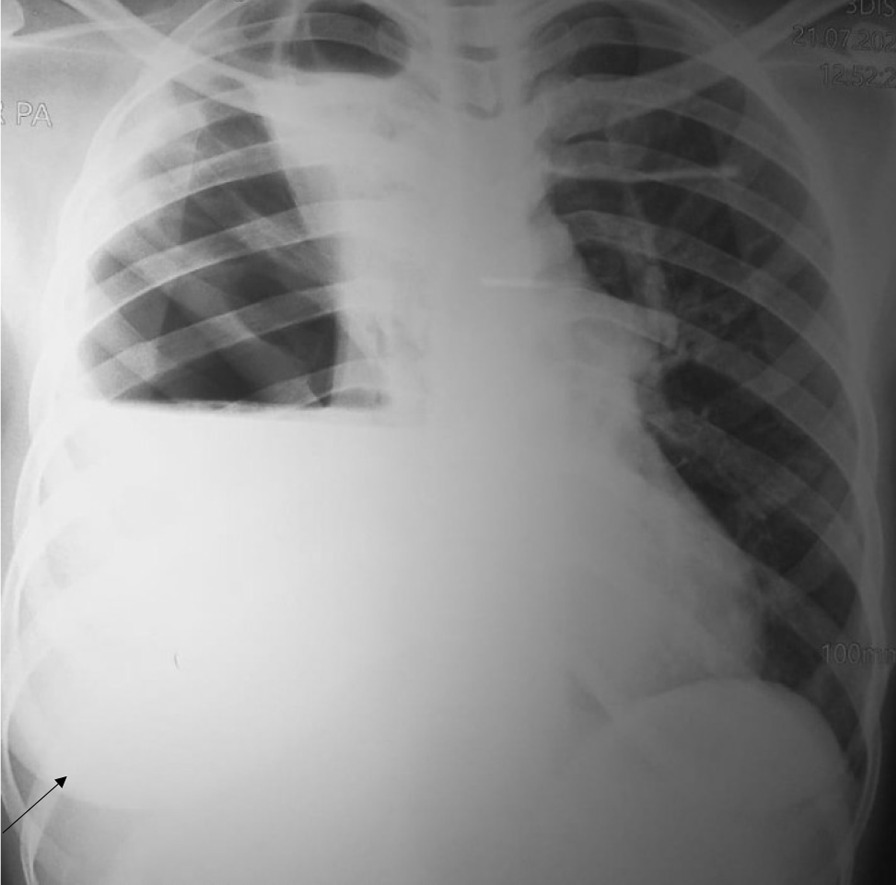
Shows a chest X-ray of right haemothorax in a participating 32 year old male
